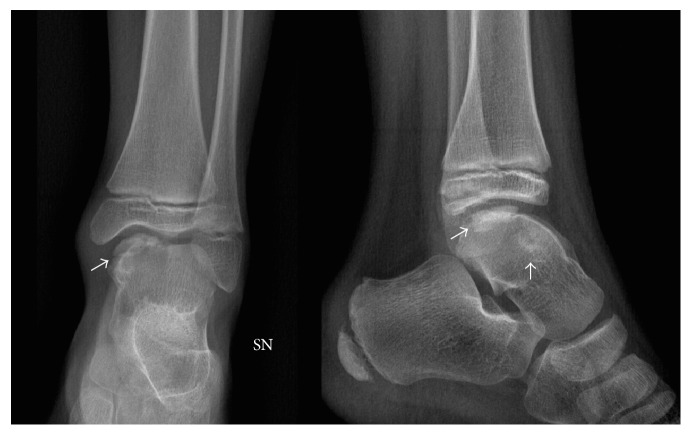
The X-rays show a bony-like protuberance localized at the anteromedial and posteromedial aspect of the talus (arrows).
